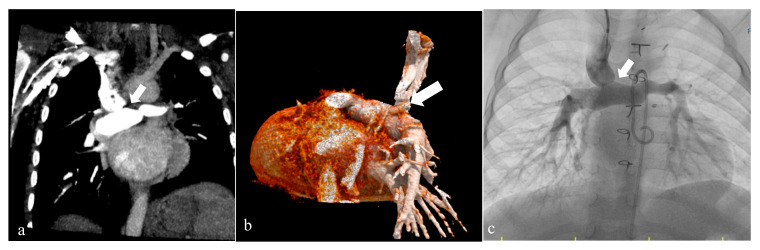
Four-year-old patient with IVS-PA and stenosis in the Glenn shunt. (a,b) Visualization of the stenosis in the Glenn shunt with CTA and 3D-CTA. (c) Visualization of the stenosis in the Glenn shunt with catheterization.
